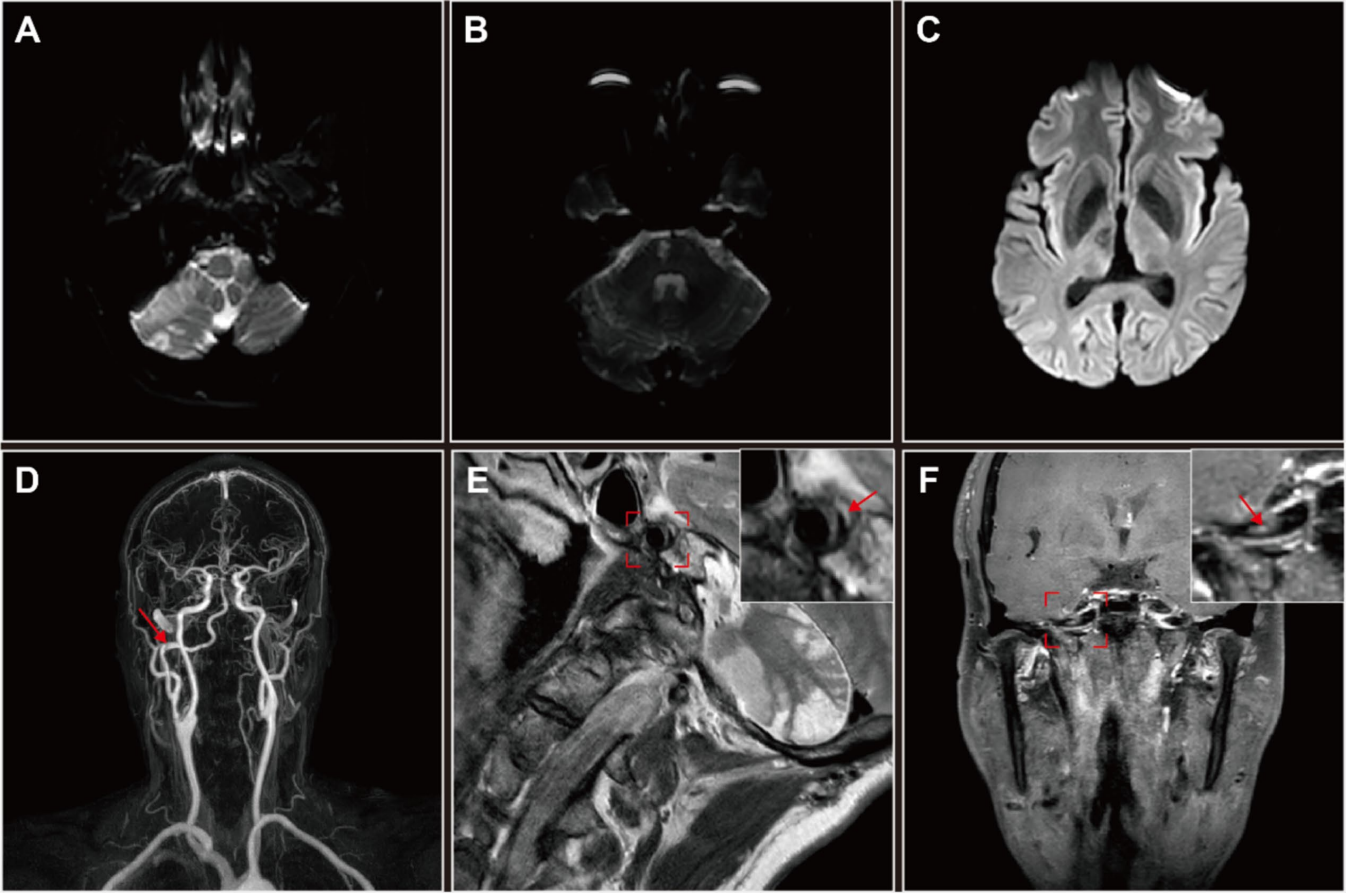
High-resolution magnetic resonance imaging (HRMRI) of the head and neck in this patient with right persistent proatlantal intersegmental artery (PPIA) dissection. (A–C) Diffusion weighted imaging (DWI) revealed multiple infarct foci and encephalomalacia across various cerebral regions: the right cerebellum (A), right brainstem (B) and right thalamus (C). (D) MRA suggested an dissection aneurysmal dilatation of the right PPIA, which is the exclusive supplier to the vertebrobasilar artery system. (E,F) High-resolution magnetic resonance vascular wall imaging (HRMR-VWI). (E) Sagittal contrast-enhanced T2-weighted imaging revealed eccentric thickening of the PPIA arterial wall accompanied by a crescent-shaped subacute-phase intramural hematoma (arrow) in the thickened lumen wall. The detailed sagittal profile of the PPIA, highlighted in a red frame, is positioned in the upper right corner. (F) Coronal contrast-enhanced T1-weighted imaging revealed inhomogeneous thickening of the intimal flap of the PPIA dissection (arrow), suggesting irregular attachment of fibrous tissue. The detailed coronal profile of the PPIA, encased in a red frame, is situated in the upper right corner. Both coronal and sagittal HRMR-VWI indicated a right PPIA dissection aneurysmal dilatation and a high risk of mural thrombosis and dislodgement.
